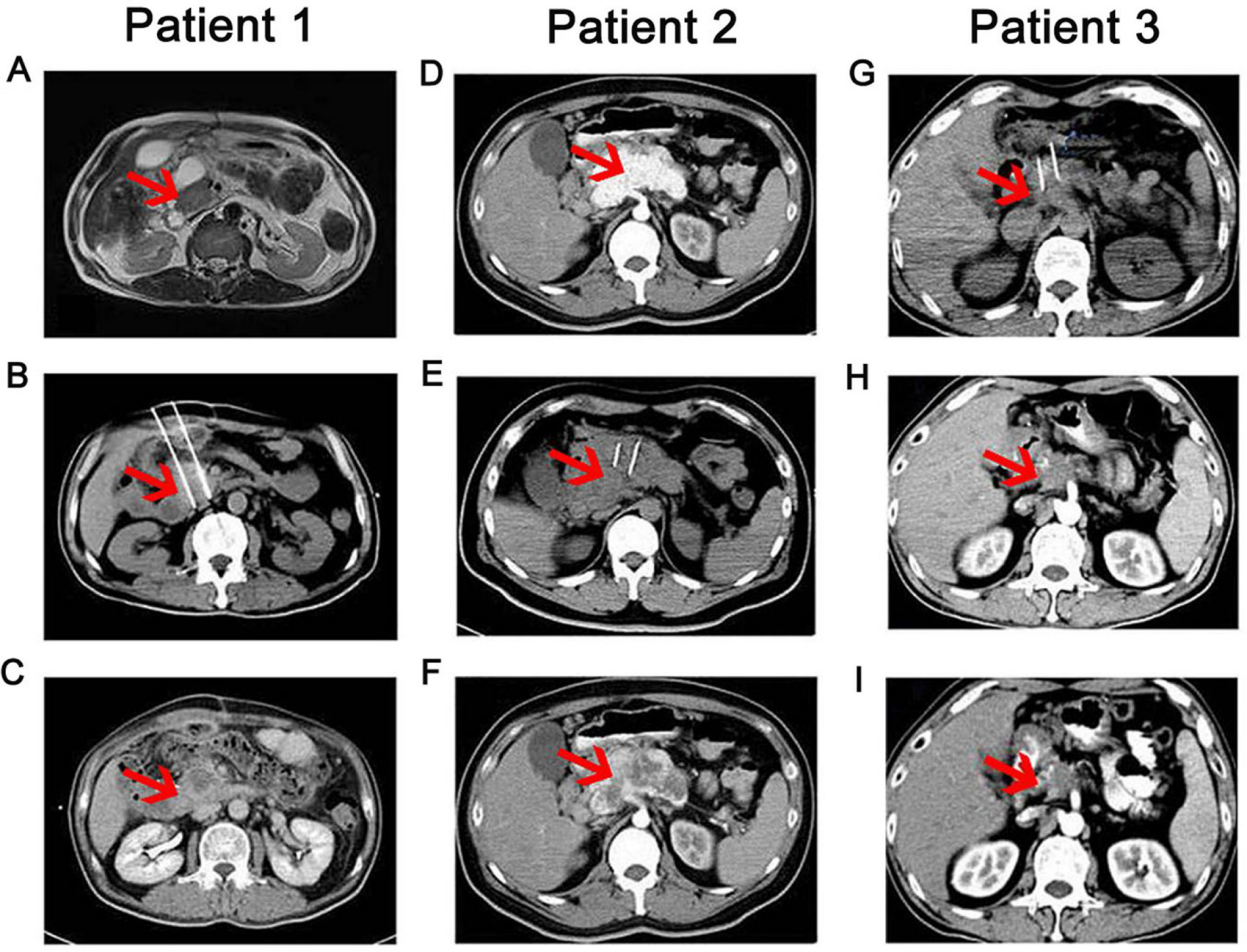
CT scans taken before, during and after IRE-NK therapy Patient 1: (A–C) (Female patient, 70 years old, T4N1M0, stage III): A. Pre-IRE CT showed a compressed duodenum and encased common bile duct and pancreatic duct due to the tumor, which was 3.1 × 2.2 cm (red arrow) in dimension; B. during IRE, two electrodes were inserted and the distance between them was 2.0 cm, as shown in this CT image; C. three month post-IRE-NK, CT showed no enhancement in the occupying lesion, with mild shrinkage of the area. Patient 2: (D–F) (Male patient, 59 years old, T4N1M1, stage IV): D. A contrast-enhanced CT scan taken before IRE showed a 5.7 × 4.2 cm contrast-enhanced lesion (red arrow) in the neck and body of the pancreas; (E) Two IRE electrodes were inserted into the tumor; F. CT scan taken two months after IRE-NK showed a 5.7 × 4.0 cm lesion with a large area of necrosis in the neck and body of the pancreas. Patient 3: (G-I) (male patient, 51 years old, T4NxM0, stage III): G. pancreatic head carcinoma, tumor size was about 4.1 × 3.5 cm (red arrow) and performed IRE ablation; H. 2 months after IRE, the tumor was reduced to 3.3 × 2.4 cm; I. 3 months after IRE, the tumor was reduced to 2.4 × 1.8 cm.
